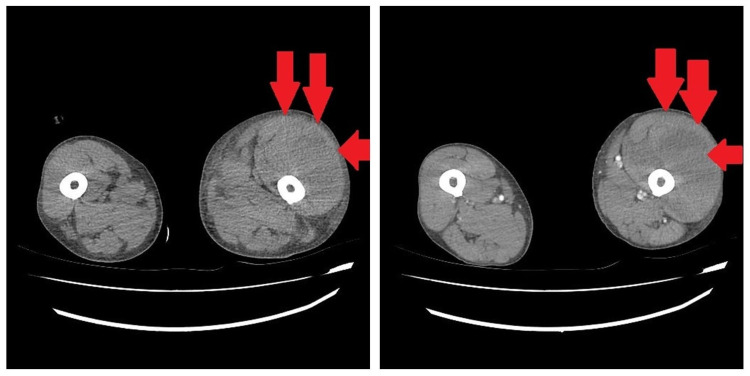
Extensive hematoma (red arrows) on the anterior-lateral surface of the left thigh (without an image of active extravasation) revealed on a lower extremity CT angiography.
